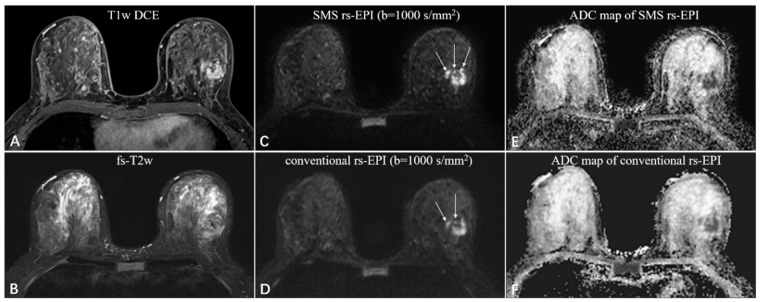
A 46-year-old woman with invasive ductal carcinoma in the left breast. The irregular mass with heterogeneous enhancement and multiple small foci around on the T1-weighted DCE images (A), and equal-to-low signal intensity on the fat-saturated T2-weighted images (B). The SMS rs-EPI image (C) shows better sharpness of anatomical details (pointed by long white arrows) and less anatomic distortion than rs-EPI image (D). The ADC signal is obviously decreased in both sequences (E,F).
